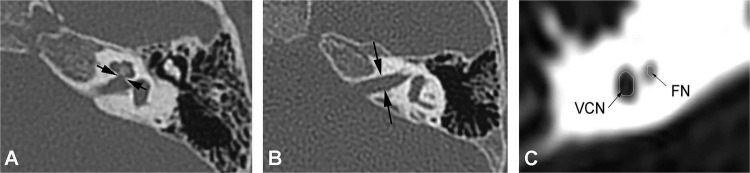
(A) Measurement of the bony cochlear nerve canal diameter using high-resolution computed tomography (distance between the two black arrows). (B) Measurement of the internal auditory canal diameter using high-resolution computed tomography (distance between the two black arrows). (C) Measurement of the area ratio of the vestibulocochlear nerve to the facial nerve at the cerebellopontine angle using magnetic resonance imaging (black arrows).
